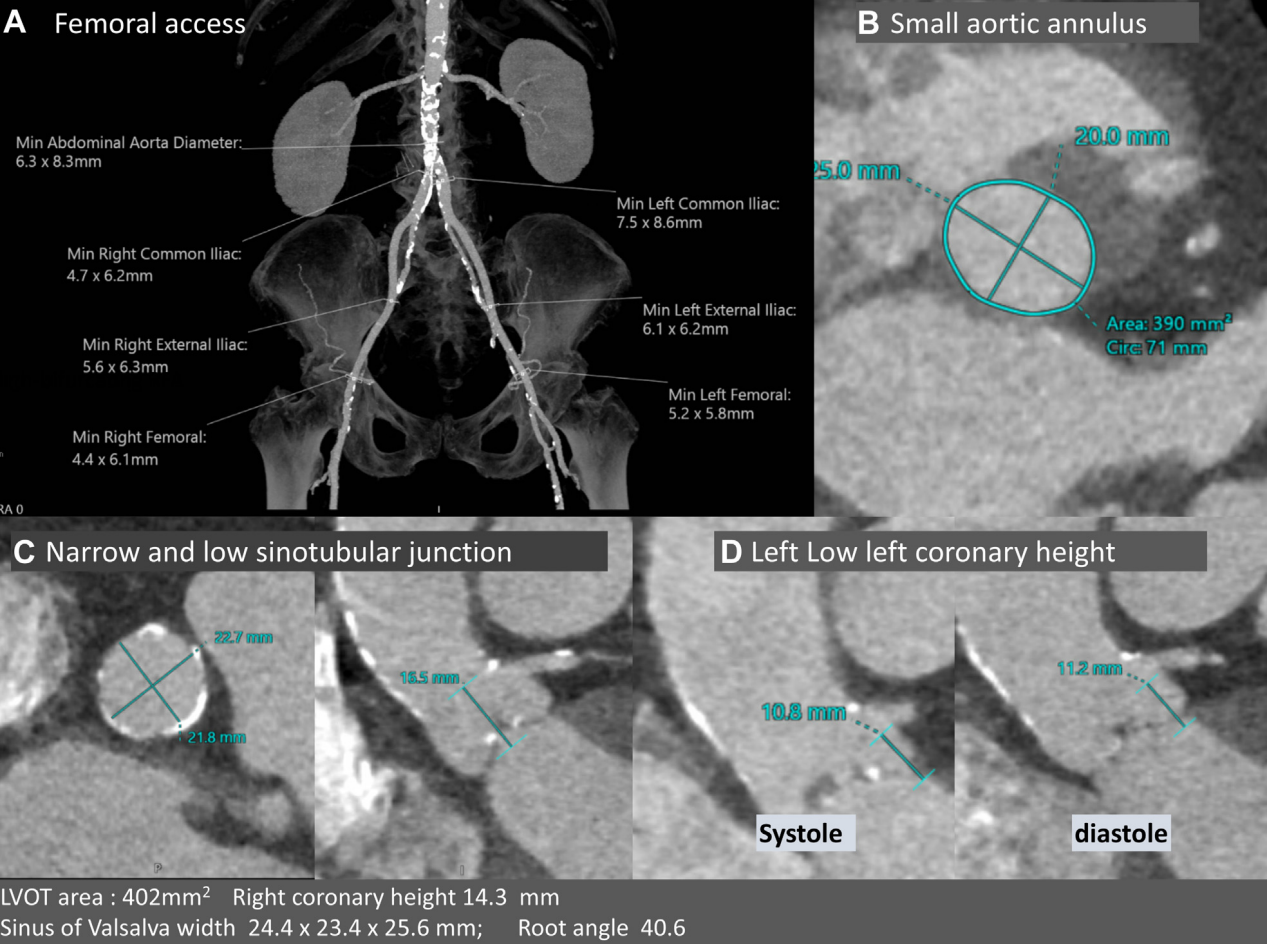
Access and Computed Tomography Workup (A) Adequate femoral access. (B to D) Annulus, sinotubular junction, and left coronary height. LVOT = left ventricular outflow ract.
